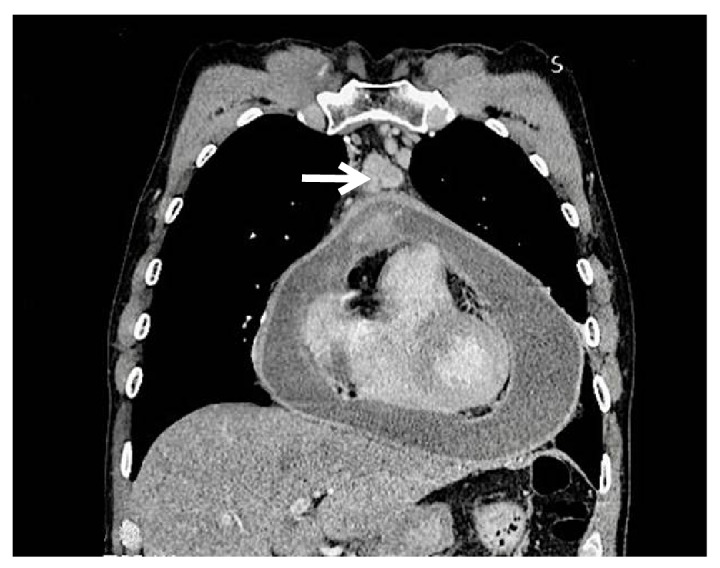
Contrasted chest computed tomography, mediastinal window. There is a 3-millimeter thickening and abnormal pericardial enhancement, pericardial effusion, which does not produce compression of the right ventricle, and pre-aortic adenopathy with heterogeneous enhancement (white arrow).
